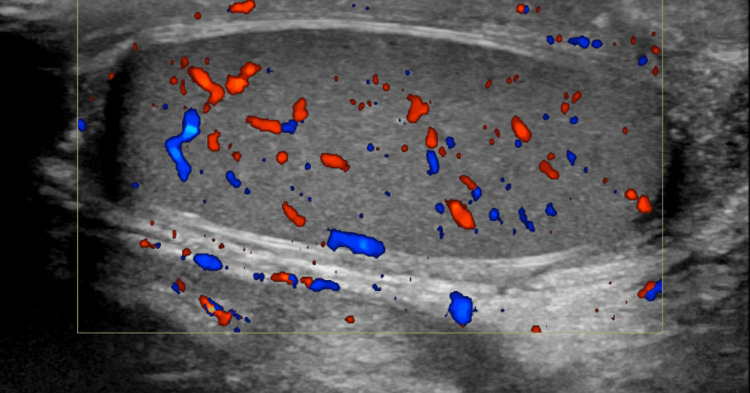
Ultrasonography of the right testes seven weeks post-injury showing normal findings with resolution of inflammation.
